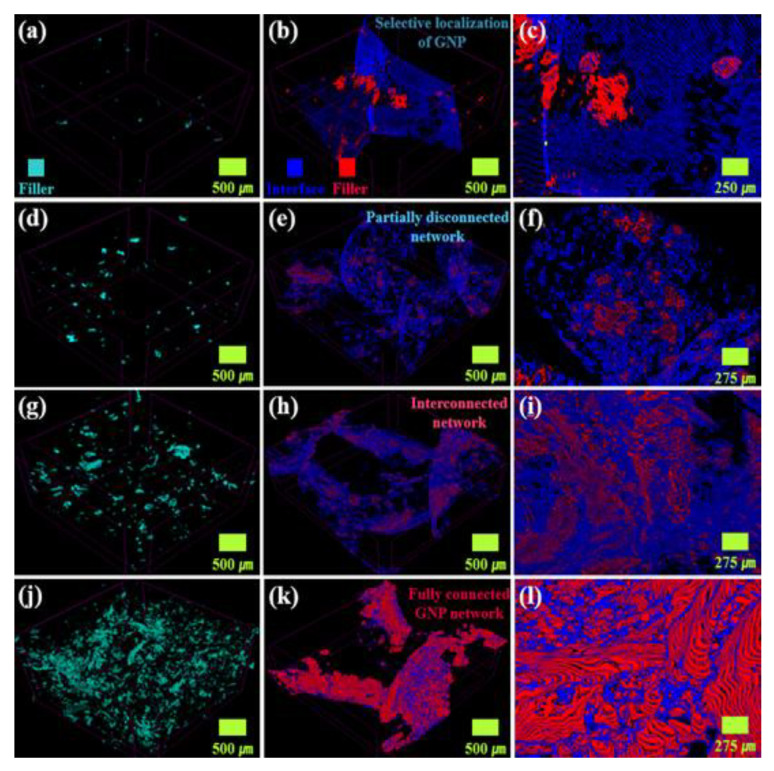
μ-CT images of (a) R-CPC0.3 and S-CPC0.3 at (b) low and (c) high magnifications, (d) R-CPC1 and S-CPC1 at (e) low and (f) high magnifications, (g) R-CPC2 and S-CPC2 at (h) low and (i) high magnifications and (j) R-CPC10 and S-CPC10 at (k) low and (l) high magnifications.
